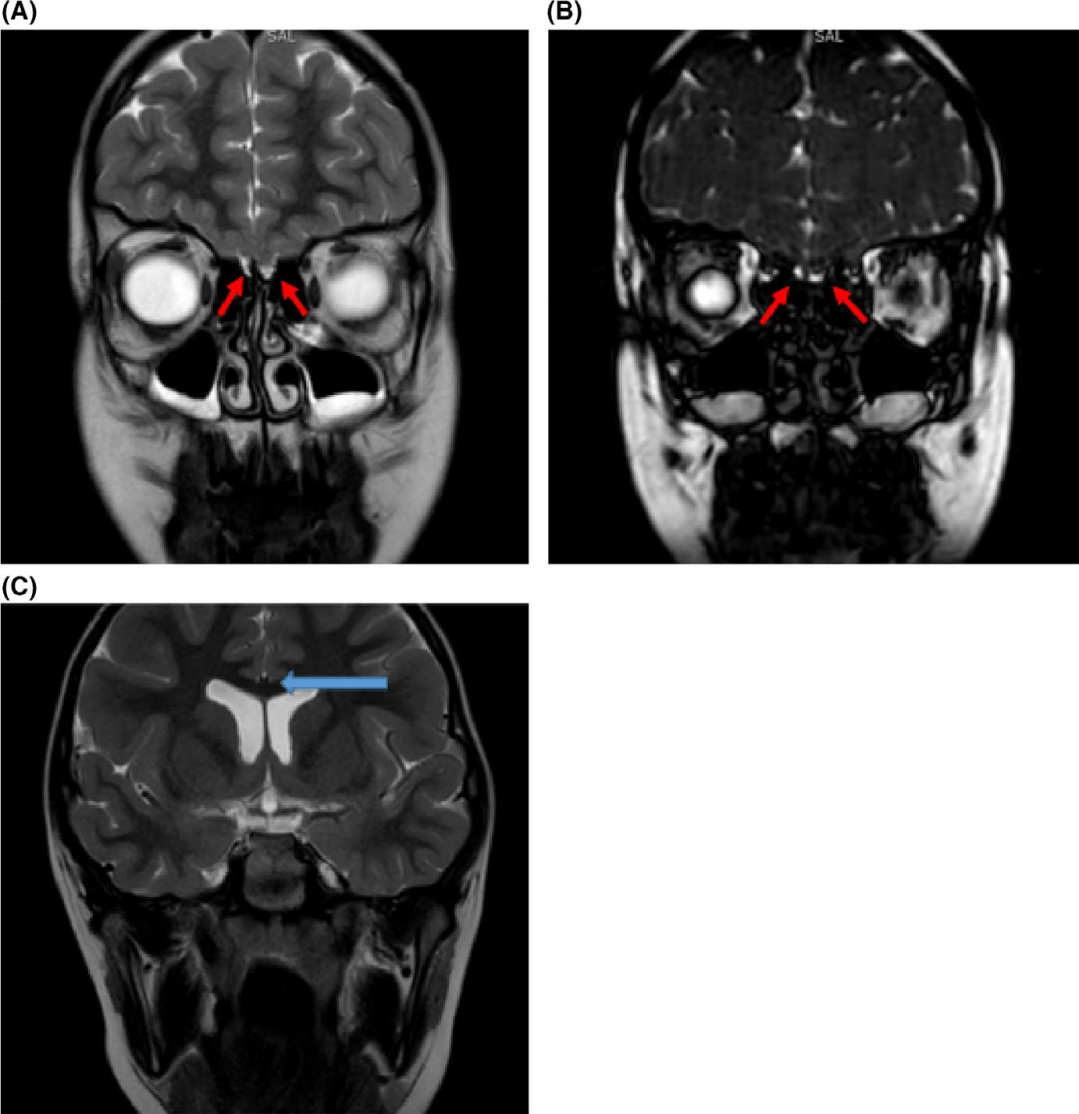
A, (T2‐weighted MRI), B, (high‐resolution T2‐weighted MRI) images, showing bilateral absence (aplasia) of the olfactory nerve with preserved olfactory sulci (Red arrows). C, (T2‐weighted MRI) showing preserved septum pellucidum and corpus callosum with no dysgeneses of posterior fossa structures (Blue arrow)
